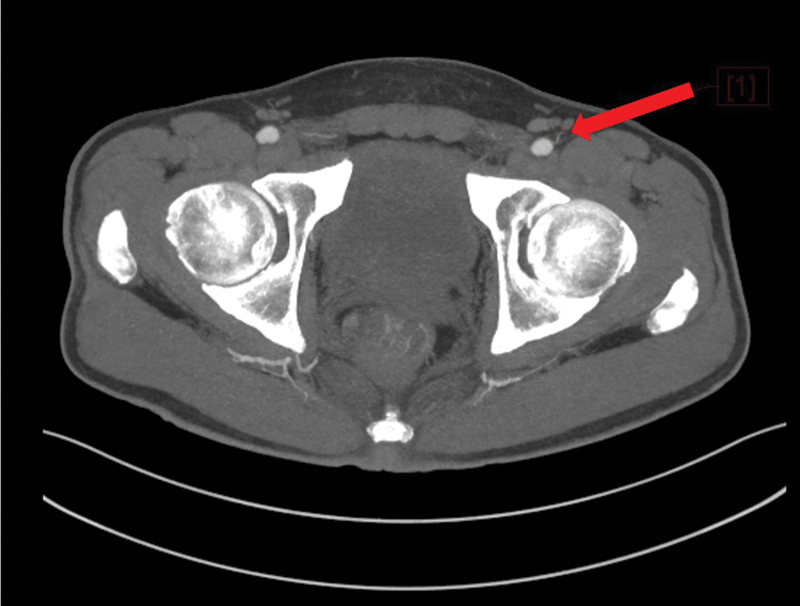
Computed tomography angiography. The red arrow shows the superficial circumflex iliac artery, with a deep and a superficial branch.
